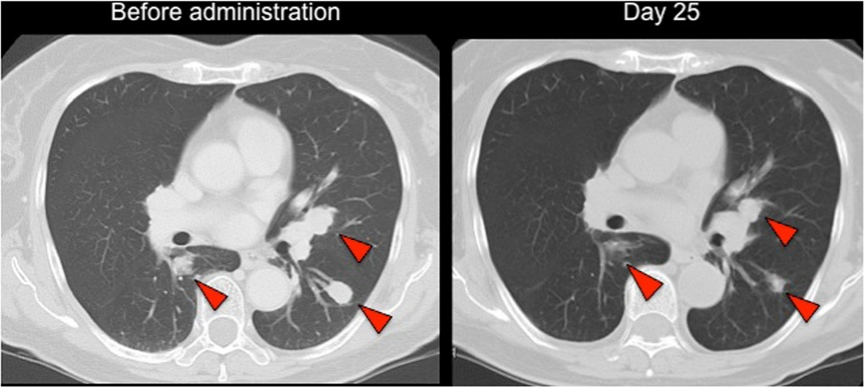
CT images. Computer tomography images before and on day 15 of sunitinib administration revealed that the size of the lung metastases was remarkably decreased by sunitinib treatment. Each arrowhead represents lung metastases.
